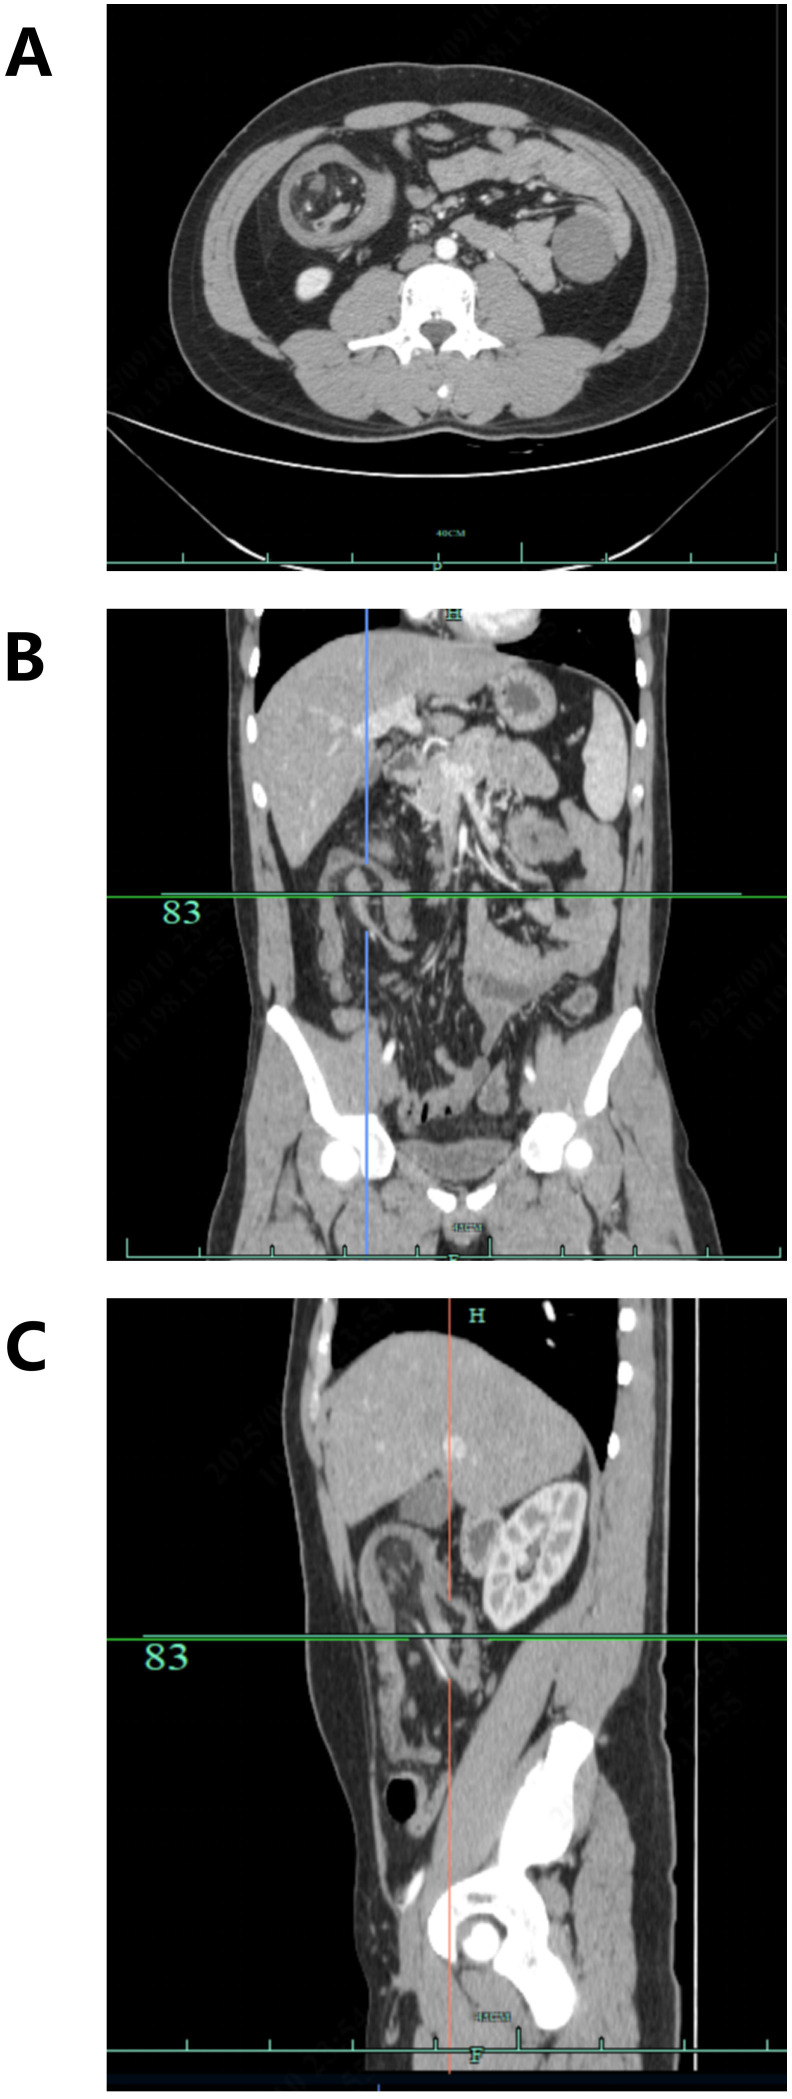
Axial (A), coronal (B), and sagittal (C) contrast-enhanced arterial-phase abdominal CT images demonstrating ileocecal intussusception in the affected patient.
